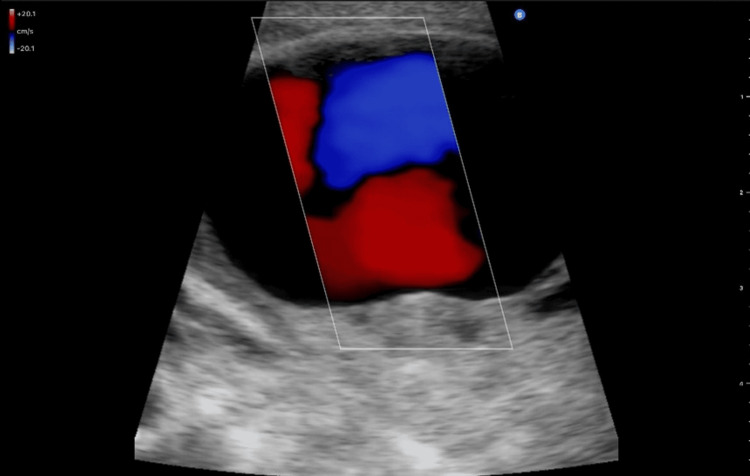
DUS overlying the left supraclavicular mass. Note the bidirectional flow with the "to-and-fro" sign. DUS: duplex ultrasound
